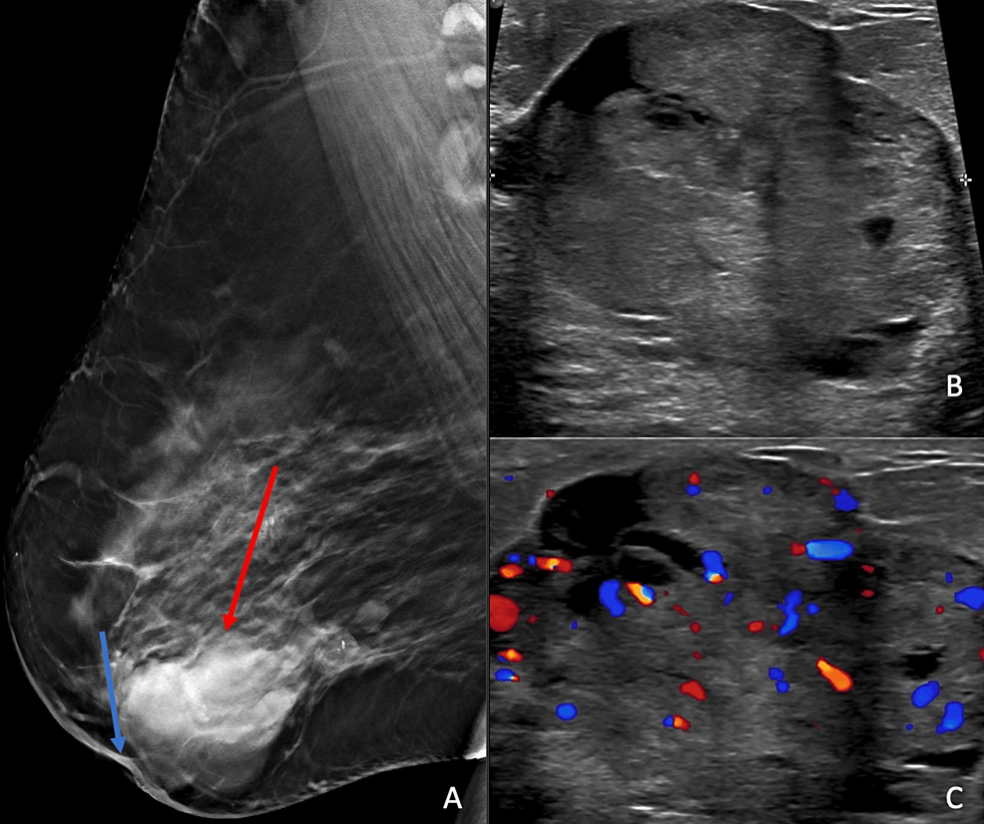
A: Right breast mammogram a year later shows skin thickening, new nipple retraction (blue arrow), and a mass (red arrow); B and C: Ultrasound of the right breast revealed a corresponding hypervascular, hypoechoic mass
